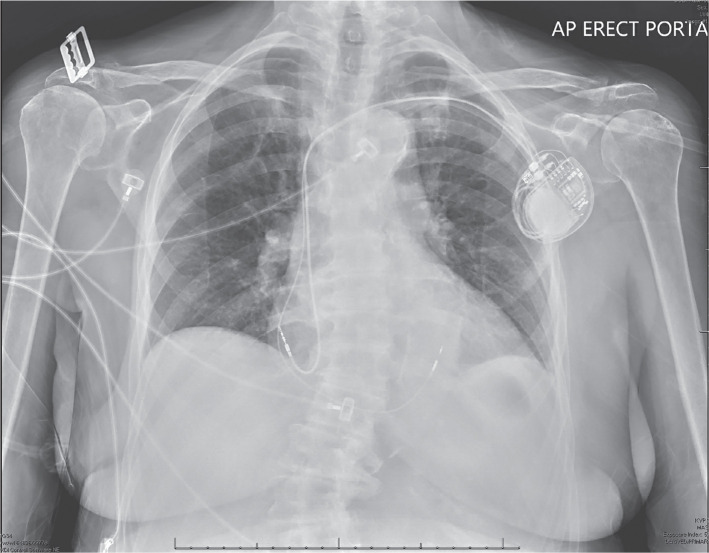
Chest X-ray showing ventricular lead position in the left bundle branch area.
